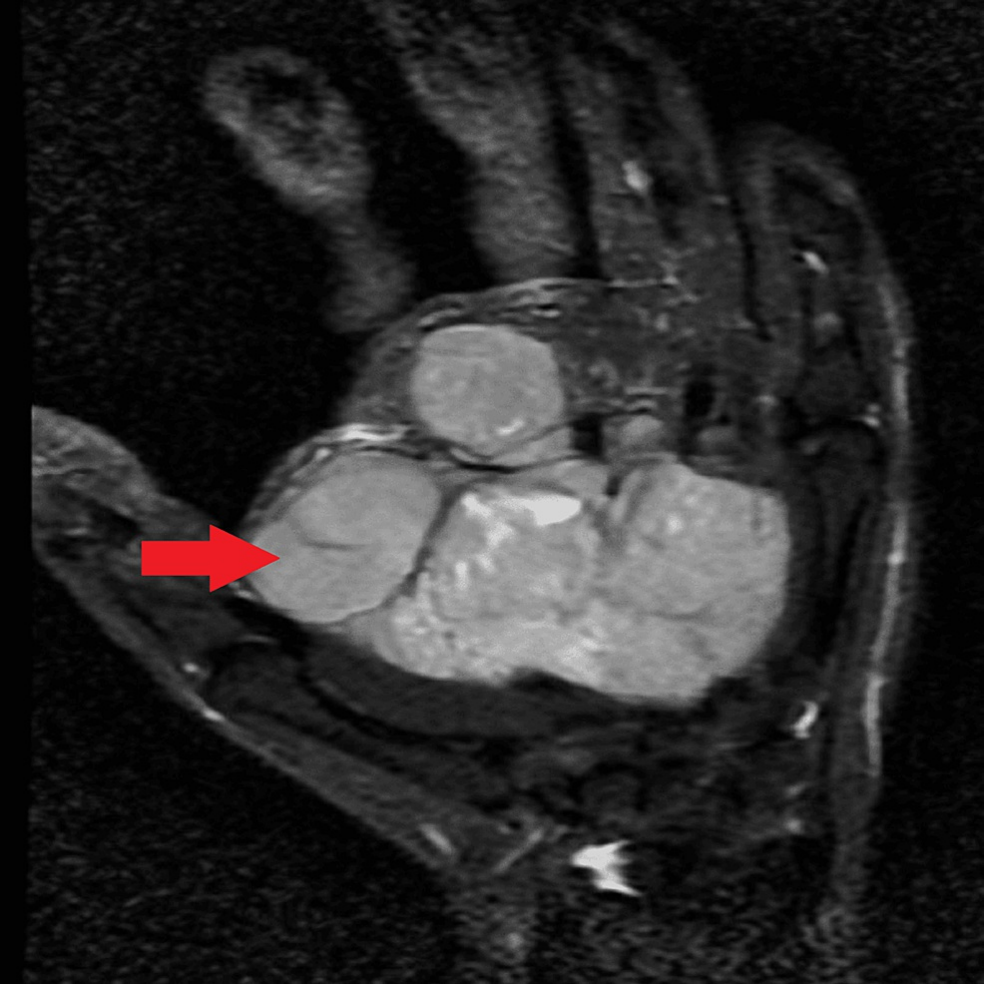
T2 weighted image of MRI MRI= Magnetic resonance imaging
